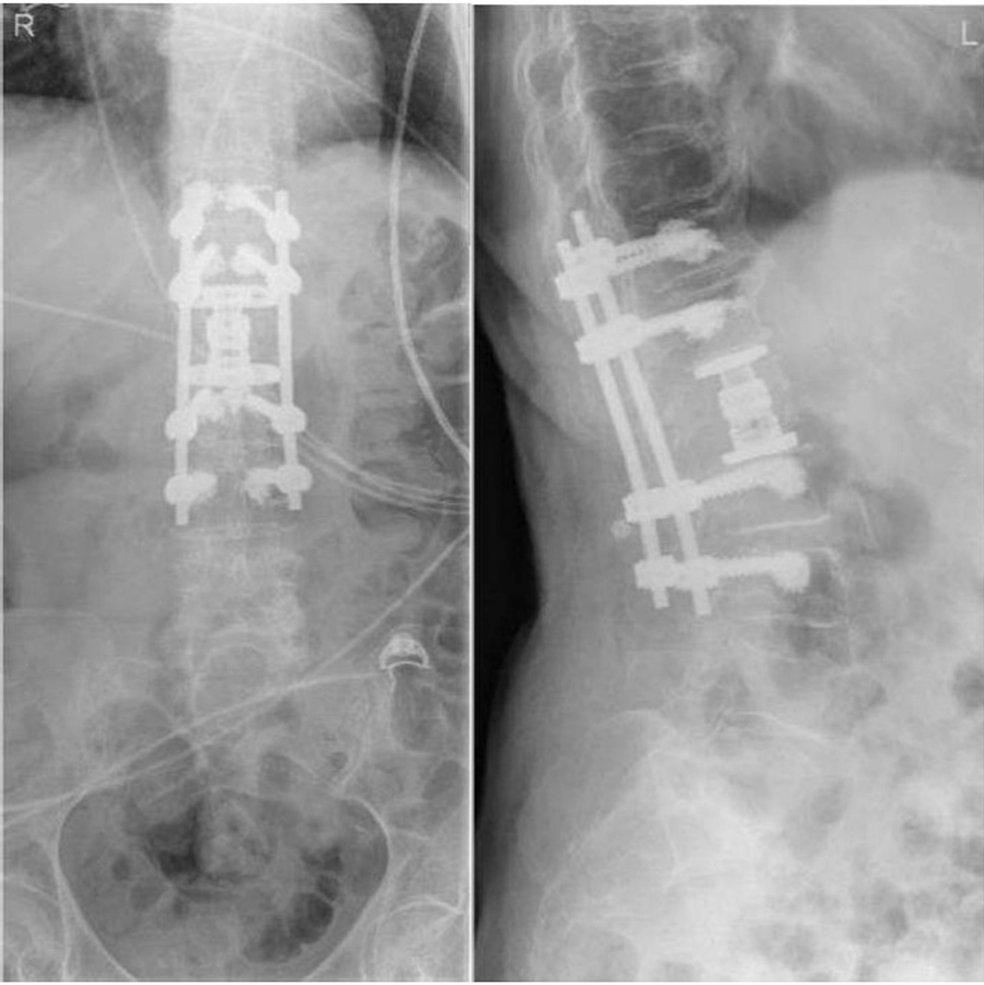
Postoperative radiographs of the lumbar spine
